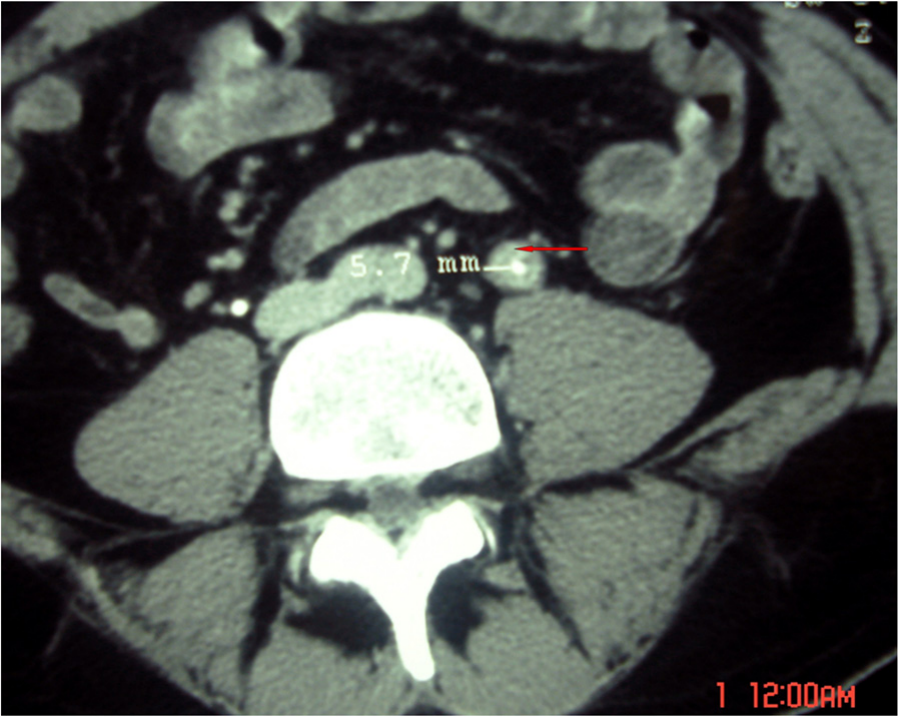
The computed tomography scan showed thickening of the tumor aspect in the left lumbar ureter (red arrow) of tuberculous origin.
